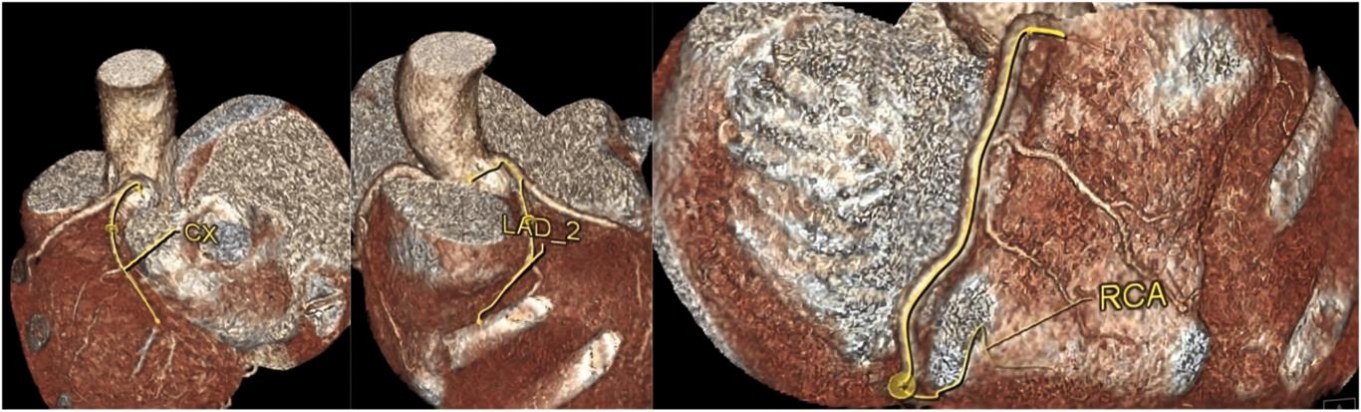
Coronary computed tomography angiography of the patient. As it was shown in the coronary computed tomography angiography, there was no stenosis observed in the left main coronary artery, left anterior descending artery, circumflex artery, or right coronary artery. CCTA, coronary computed tomography angiography; CX, circumflex artery; LAD, left anterior descending artery; RCX, right coronary artery.
